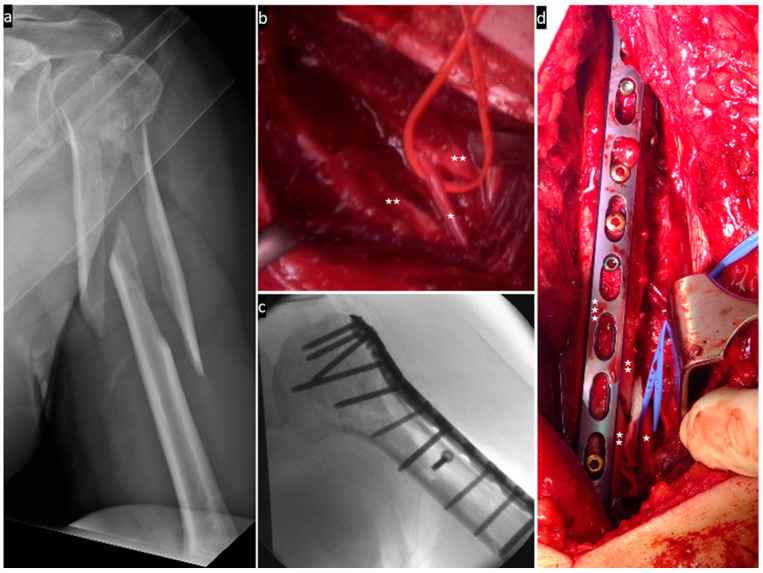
(a) Preoperative X-ray of the proximal fracture; (b) the nerve (one asterisk) is interposed between the bone fragments (two asterisks); (c) intraoperative X-ray control; and (d) the nerve (one asterisk) is now neurolysed and separated from the fracture (two asterisks) stabilised with a locking plate (three asterisks) by an absorbable mesh.
